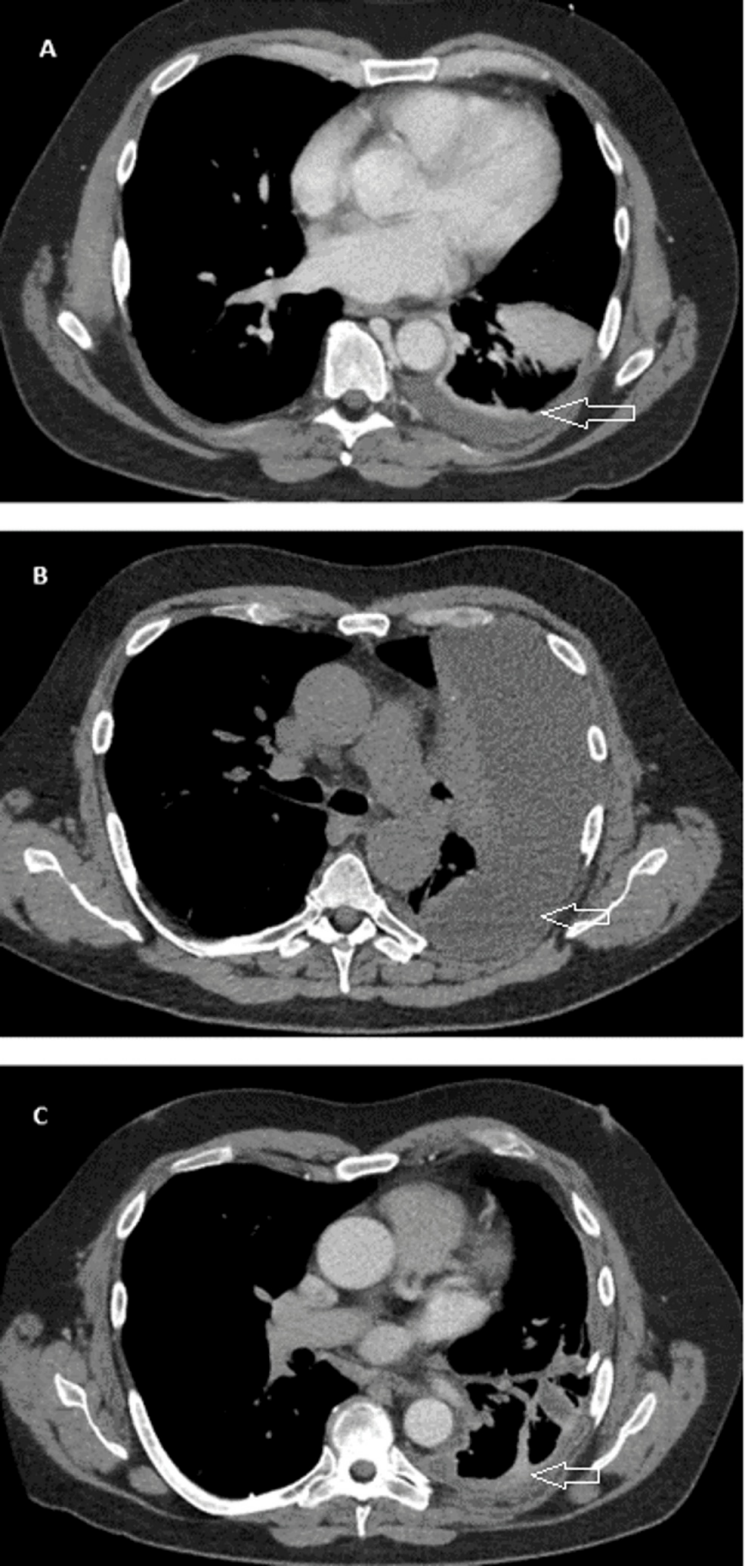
Serial CT imaging A: Day 0: left lower lobe consolidation with trace effusion. B: Day 5: development of a large effusion. C: Day 16: decreased effusion with thick septations. Arrows point to the patient's pleural effusion.
